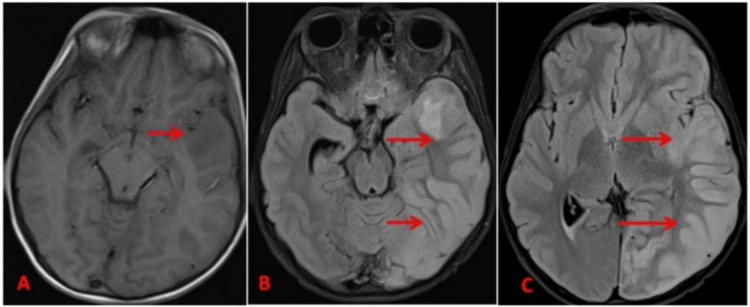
(A) Axial T1, (B-C) Axial FLAIR showing ill-defined hypointense signal in the left temporal lobe on T1-weighted images, appearing hyperintense on FLAIR (marked by red arrows). FLAIR: fluid-attenuated inversion recovery.
